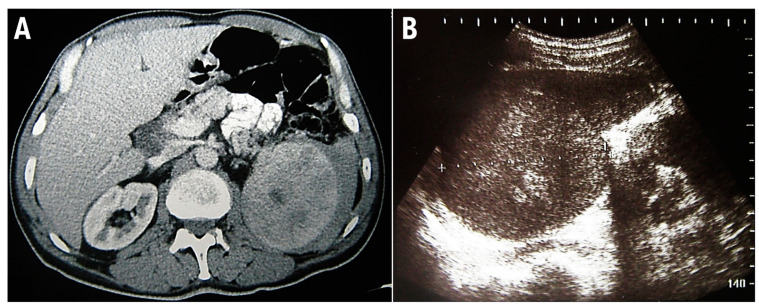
Multidetector computed tomography reveals enlarged, inhomogeneous spleen with intraparenchymal confluent zones of lower density, and a small amount of free fluid is present along the lateral contour of the spleen (A). Abdominal ultrasonographic examination shows enlarged spleen with diffusely inhomogeneous parenchyma (B).
